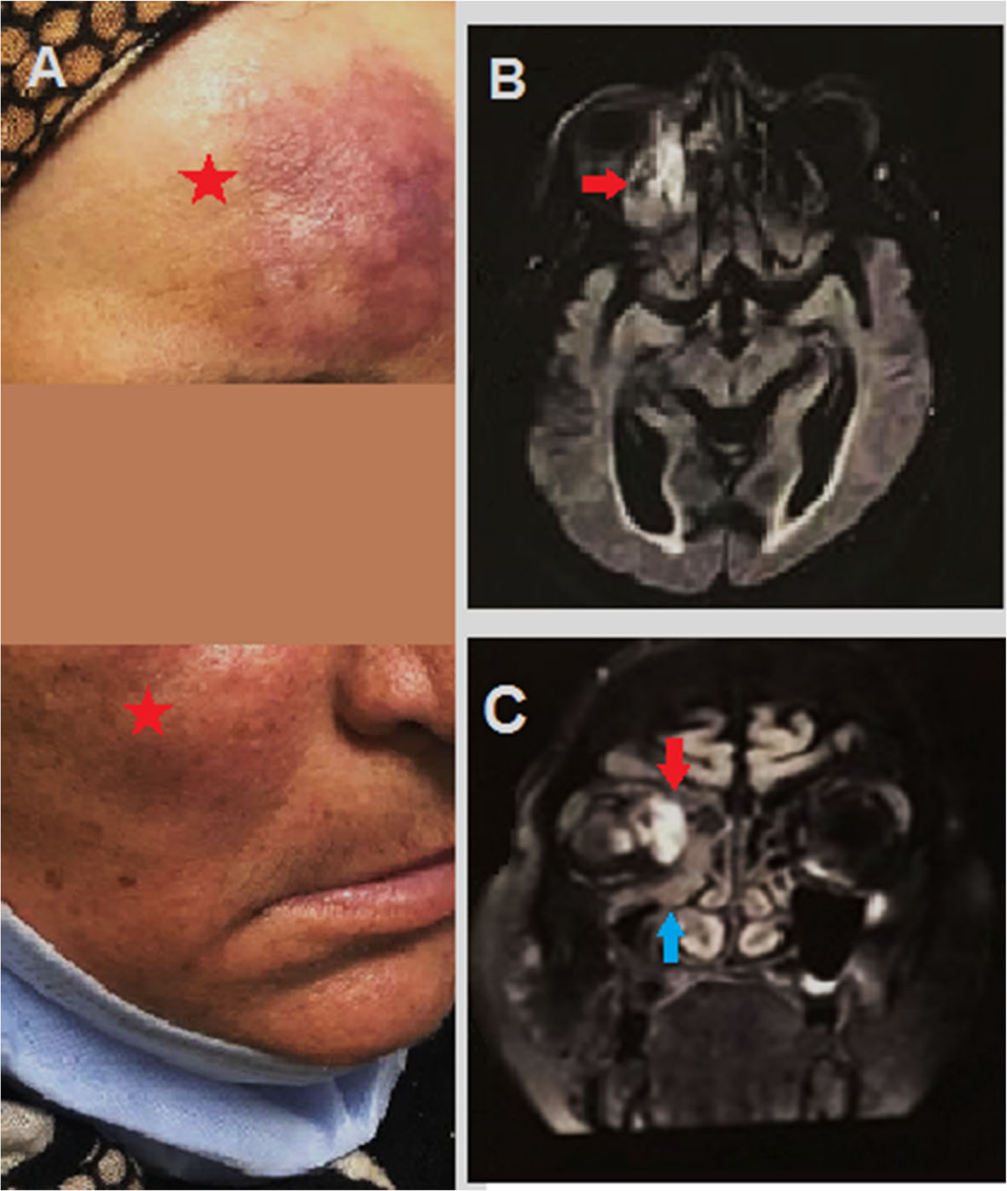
A Red star highlighting elevated dark colored forehead and right cheek that goes with cutaneous form of mucormycosis. B Red arrow along axial MRI with obliteration of right ostiomeatal complex. C Red arrow along coronal MRI revealing obliteration of right ostiomeatal complex with infiltration of infection to the right orbital cavity. Blue arrow showing maxillary, ethmoid and frontal sinusitis all around the right orbital cavity
